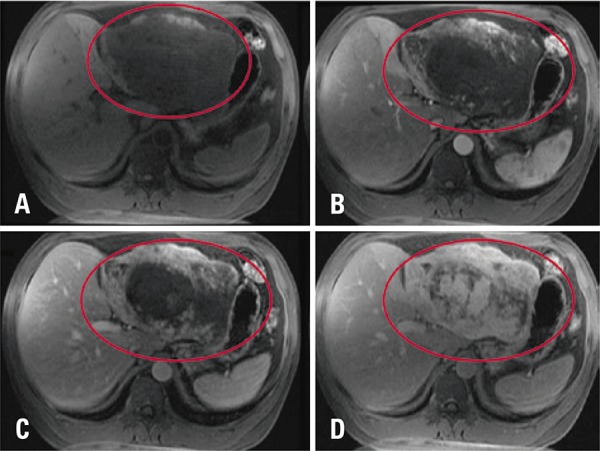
Typical giant hemangioma. Axial sequences of the magnetic resonance in T1-weighted image pre- (A) and post-contrast arterial (B), portal (C) and delayed (D) phases, showing giant hemangioma in segments II and III, with peripheral and discontinued globuliforme-enhancement, and tendency to homogenization
